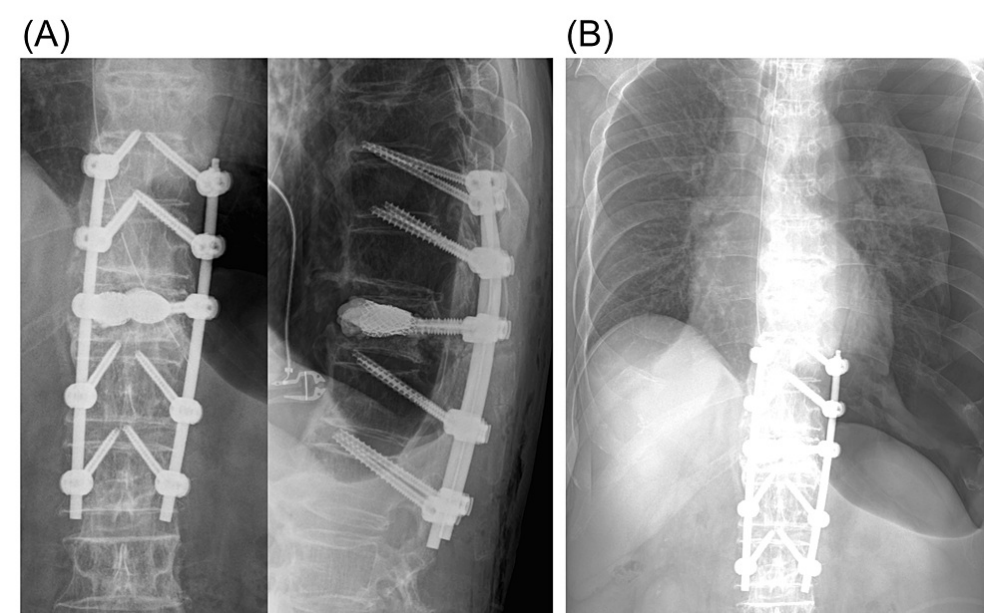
Postoperative x-rays of the patient. The images show (A) kyphoplasty at T12 and T10-L2 posterior fixation using PES was performed by the percutaneous technique. (B) Pneumothorax at the left side could be observed in the postoperative radiograph by adjusting the window level to the lung condition. PES: penetrating endplate screw
